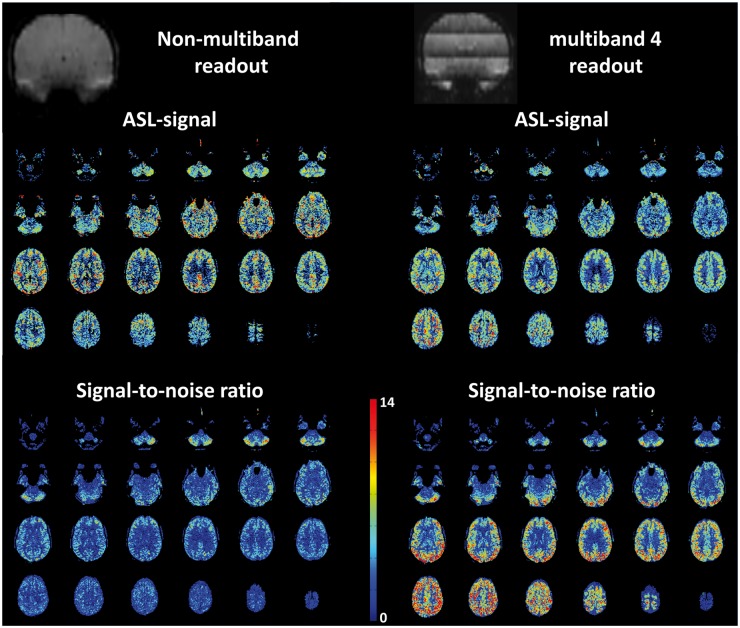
Example of the potential of multiband (also known as simultaneous multi-slice (SMS) acquisition) for ASL (scans acquired on a 3 T MRI scanner (Ingenia, Philips, The Netherlands; scanner software was locally adapted to allow multiband ASL) on a 43-year-old, male volunteer who provided informed consent under an approved IRB-protocol for protocol development). Left: traditional readout with single-shot EPI (half Fourier of 0.8; readout time per slice 60 ms; 24 slices; 1800 ms labelling duration; 1800 ms PLD; two background suppression inversion pulses; 30 averages; total scan time 5m08s). Right: same settings except for multiband factor of 4; 38 averages; total scan time 5m11s. Upper row: coronal reconstruction of mean control scans showing a gradual decrease in background suppression efficiency for the traditional readout (Left) and four bands of decreasing background suppression efficiency for the multiband-4 readout (Right). Middle row: ASL-images. Lower row: SNR images calculated as ASL-signal divided by standard deviation over the acquired averages multiplied by the square root of the number of averages to achieve the effective SNR corrected for the shorter achievable TR for multiband readout as compared to the traditional readout.
